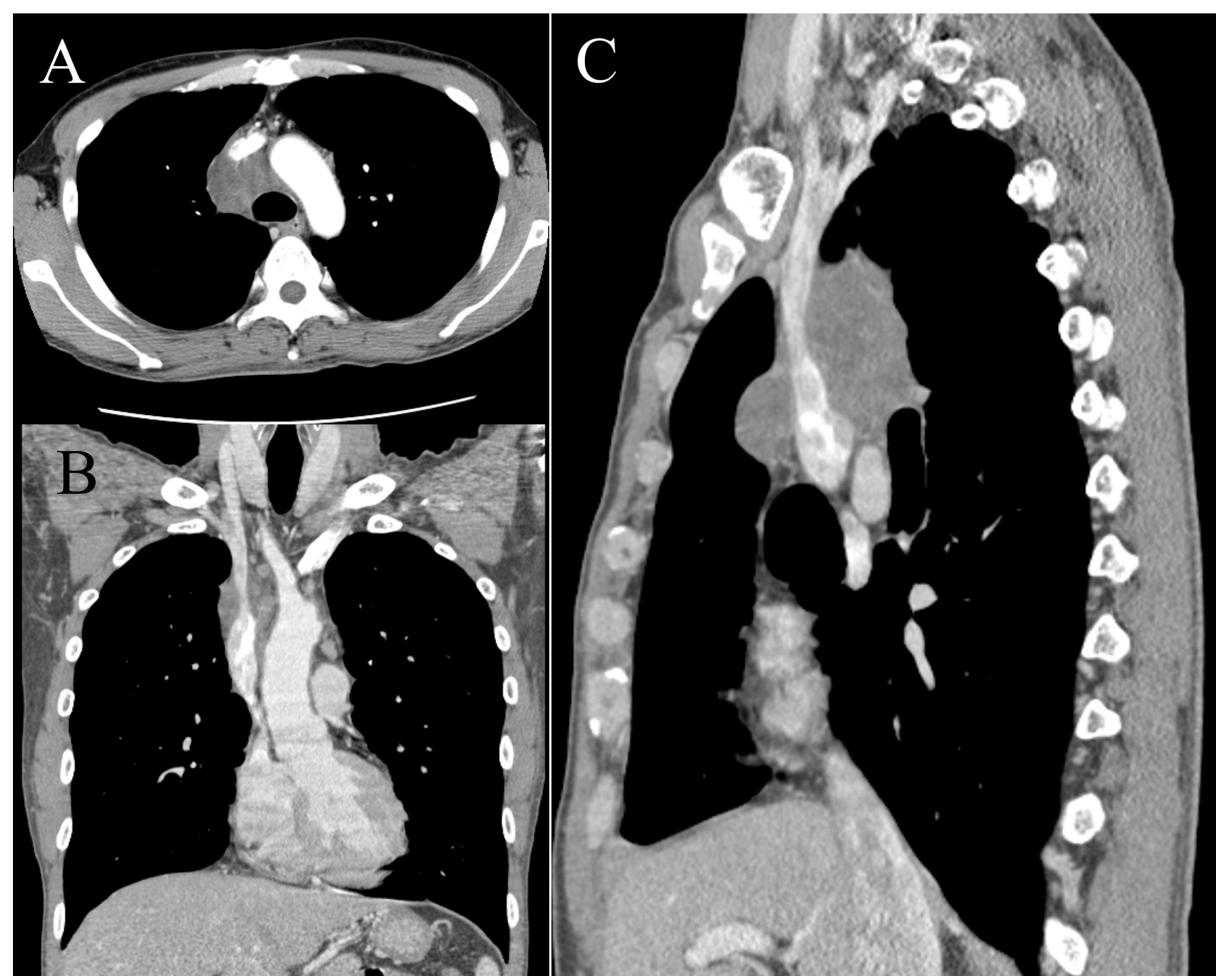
Mediastinal tumor after neoadjuvant chemotherapy (A) Axial, (B) coronal, and (C) sagittal views of computed tomography show the tumor reduced to 7 cm in maximum dimension after chemotherapy. Primarily located in the right lower paratracheal region, it extensively invaded the superior vena cava, especially posteriorly. (Pretreatment images unavailable due to expired storage period.)
